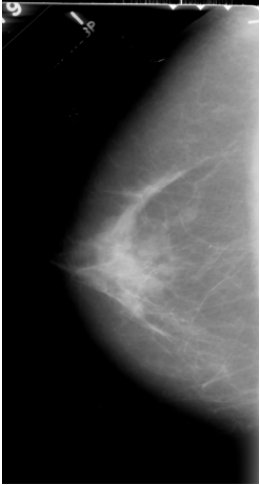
Chest X-Ray Dataset	Normal	Bacteria	Virus
